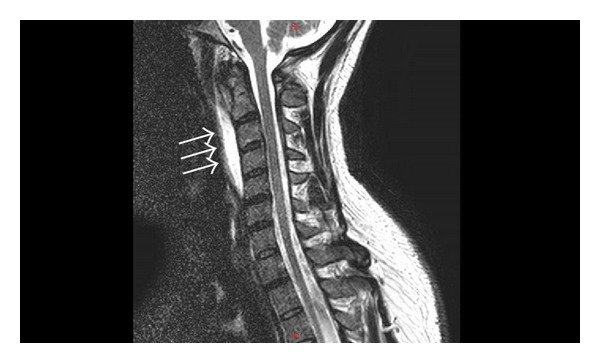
Sagittal T2-weighted MRI of the neck shows bright signal representing a prevertebral fluid collection.
